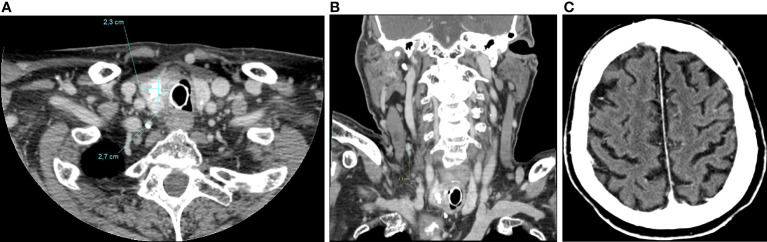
CT-scan performed in October 2022, 13 months after the beginning of treatment, showing a further tumor volume reduction of left thyroid lobe (2,3x2,7cm) (A), of latero-cervical lymphadenopathies (maximum diameter of 1,3 cm) (B), and a complete radiological response of brain metastases (C).
